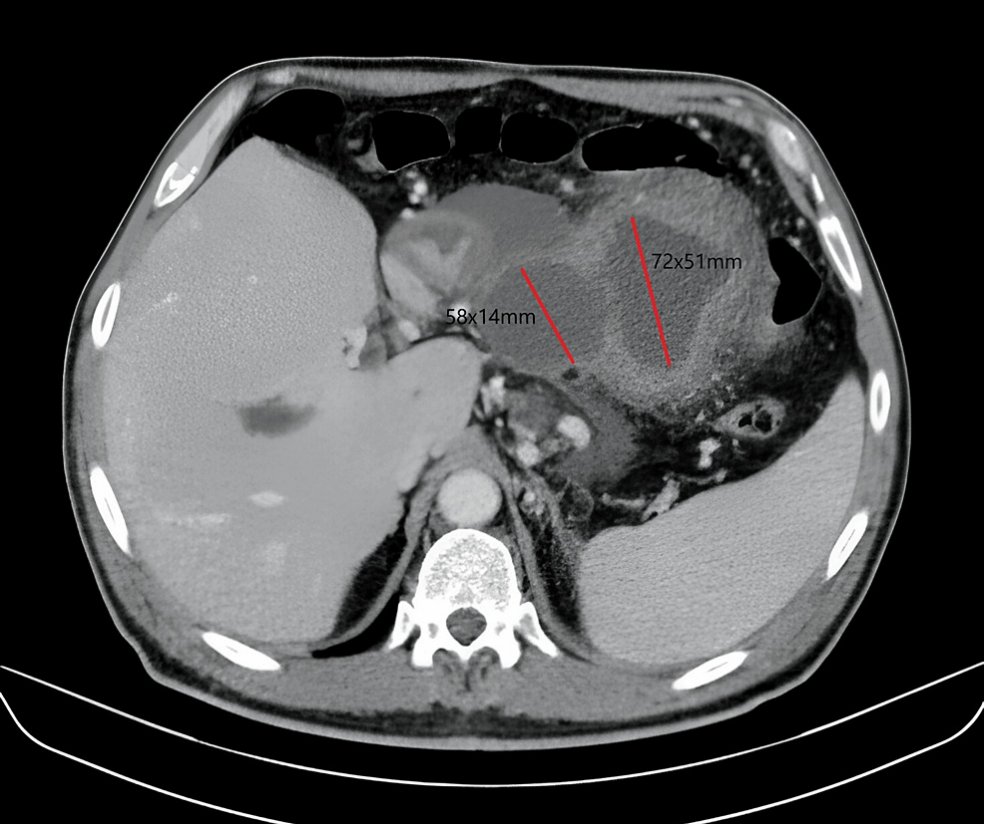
CT scan at two months There is mural thickening of the pseudocyst wall, with possible superinfection. This collection seems to be connected, in a filiform form, with another one of more medial and caudal locations measuring 72x51x56 mm. There are other peripancreatic collections of 58x14x33 mm. and other multiple ones of very small size, which do not allow visualization of the pancreatic body and tail. Wirsung's dilatation is observed in this region of the body and tail, with suspicion of the underlying neoplastic process.
